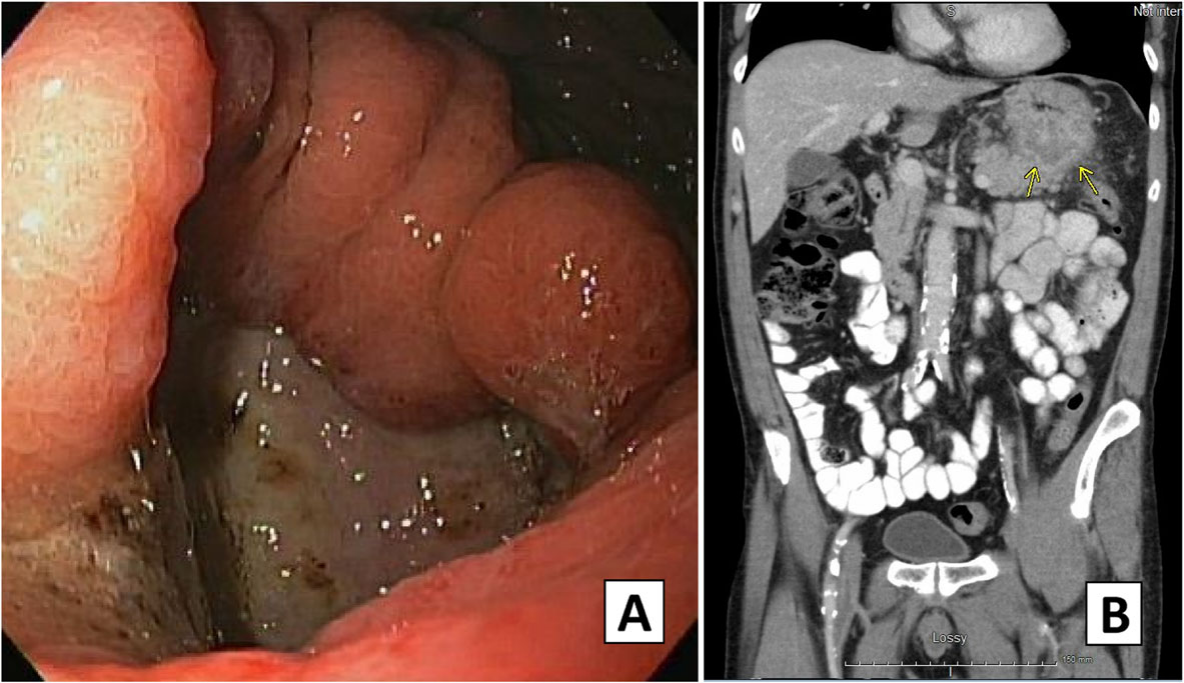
Initial endoscopic appearance of ulcerated mass in gastric fundus (a). Preoperative contrast-enhanced CT scan of the abdomen and pelvis (b). Yellow arrows demonstrate mass in gastric fundus and body
